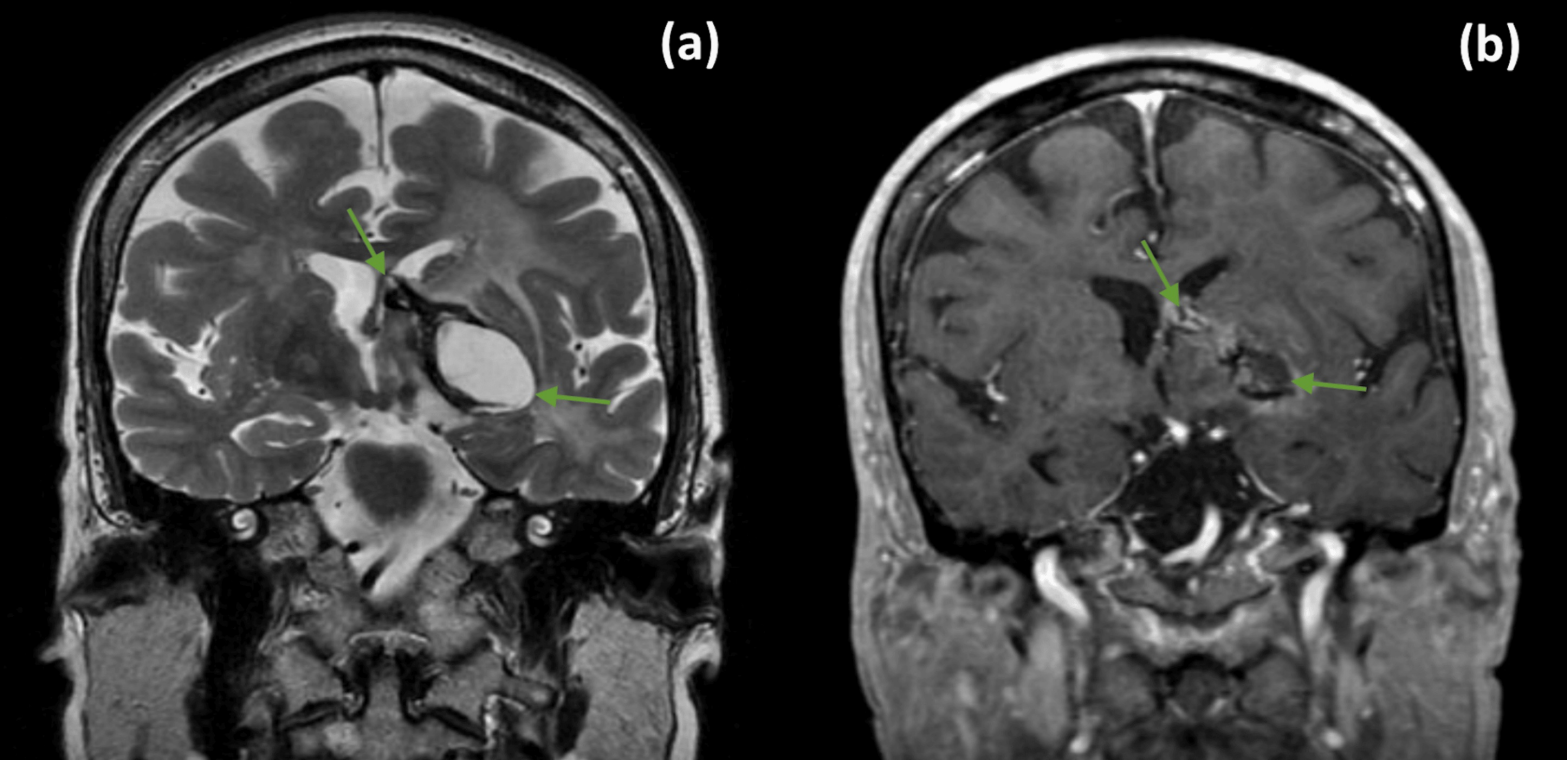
Brain MRI 6 months after RT treatment. T2-WI (a) and post-contrast T1-WI (b) revealed a reduction in tumor dimensions, particularly of the cystic component (green arrows) that extends to the body of the left lateral ventricle.
